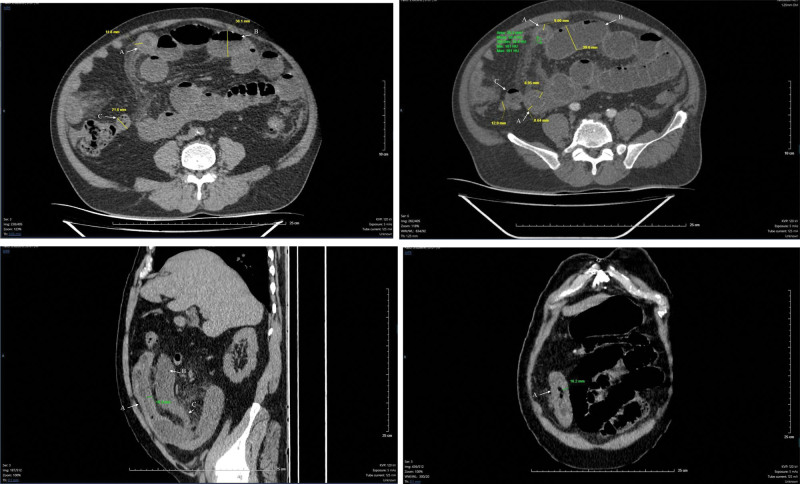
Multiplanar reconstructions. Non-contrast abdominal CT with axial multiplanar reconstruction (MPR). Segmental circumferential small-bowel wall thickening (A) with associated luminal narrowing is observed, accompanied by proximal bowel dilatation (B) and distal bowel collapse (C). Axial contrast-enhanced abdominal CT in the arterial phase (slice thickness 1.25 mm) with quantitative attenuation measurements (HU). The characteristic “target sign” (A) is clearly demonstrated, reflecting layered attenuation of the bowel wall. Quantitative attenuation measurements confirm increased intramural density. Non-contrast abdominal CT with coronal multiplanar reconstruction (MPR). The longitudinal extent of the affected bowel segment (A) is clearly delineated, with associated proximal dilatation (B) and distal collapse (C). Non-contrast abdominal CT with sagittal multiplanar reconstruction (MPR). Segmental bowel wall thickening (A) is demonstrated, clarifying the spatial orientation of the lesion along the bowel axis. CT = computed tomography, MPR = multiplanar reconstruction.
